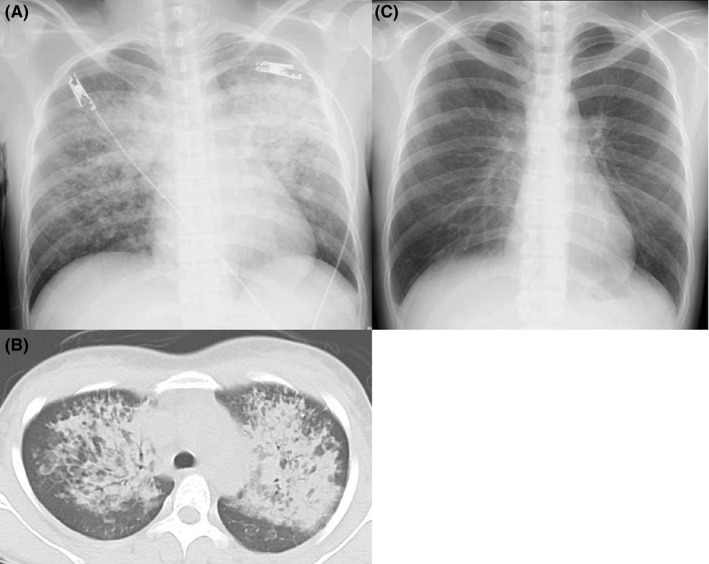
A, Chest radiography image upon admission, (B) computed tomography of the chest upon admission, (C) and chest radiography 4 d after admission
